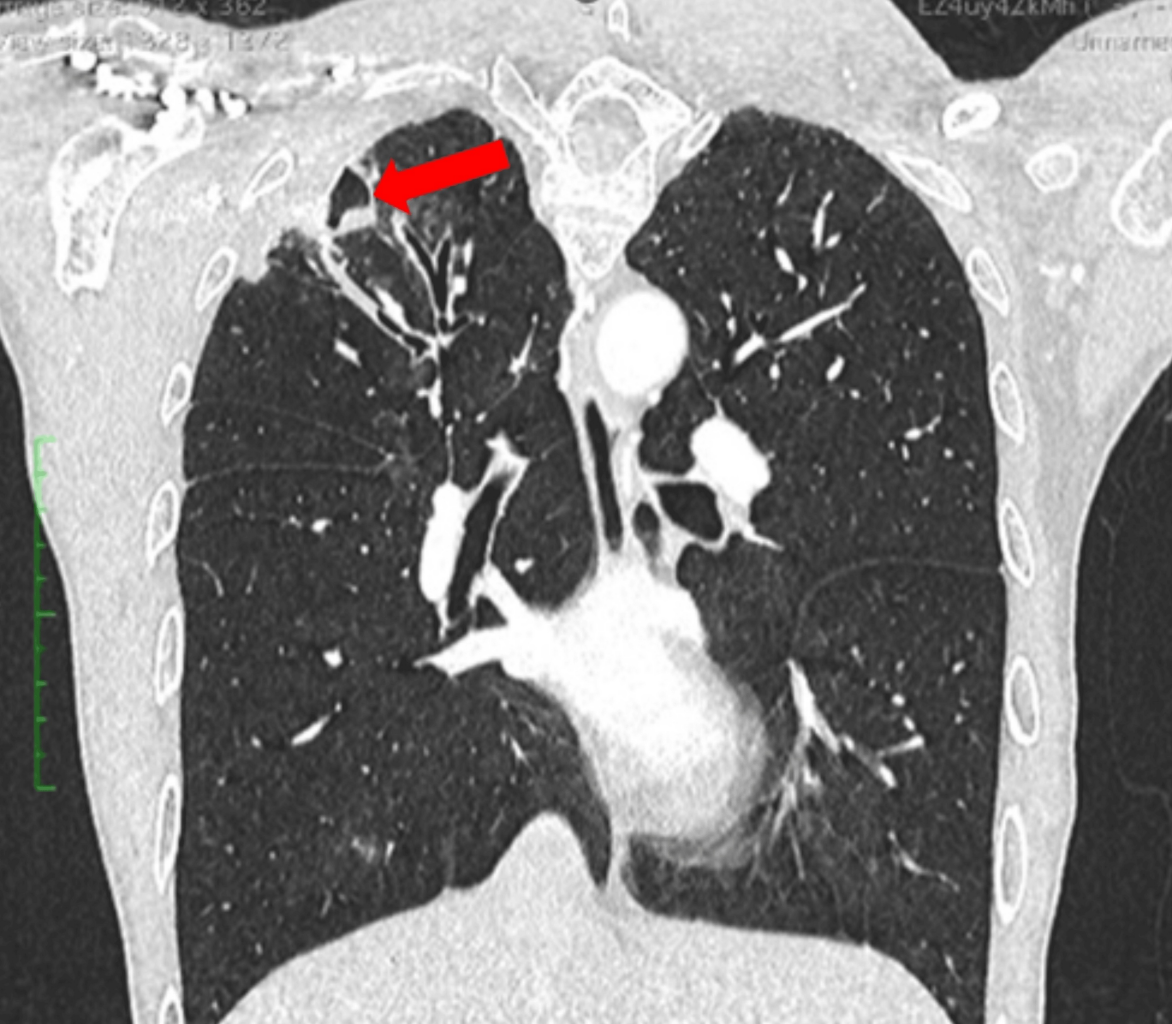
Chest CT revealed a thin-walled cavitary lesion with focal calcification in the posterolateral right apex, consistent with a chronic process Coronal CT thorax (lung window) demonstrating a right upper lobe cavitary lesion with thin wall and calcification (arrow) (case 2)
